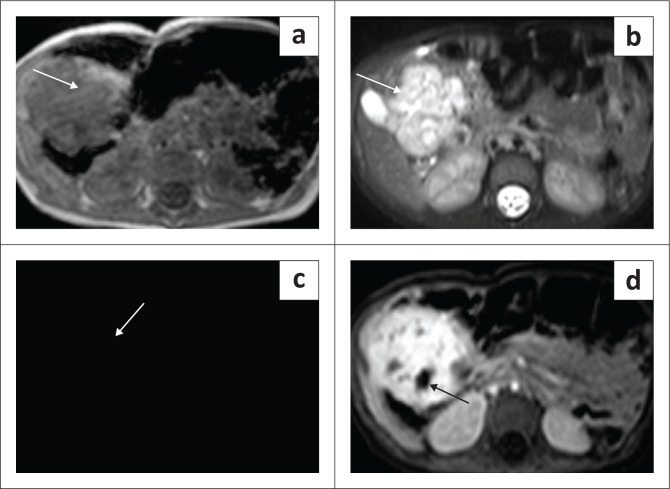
Axial T1-weighted (T1W) image (a) and axial T2-weighted (T2W) image (b) showing a well-marginated lesion, brightly T2 hyperintense, in the right lobe of liver. Dynamic contrast-enhanced axial T1W fat-saturated images (c and d) showing peripheral nodular enhancement in the arterial phase (arrow in c) with centripetal filling and almost complete enhancement with a few nonenhancing areas (arrow in d). The above features are typical for a solitary infantile hepatic haemangioma.
